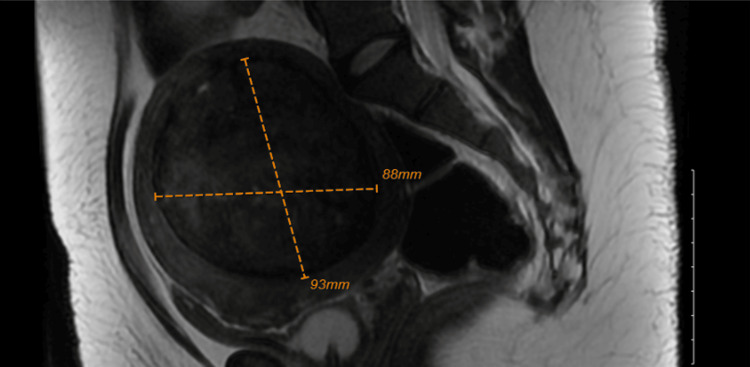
MRI pelvis demonstrating largest intramural fibroid
